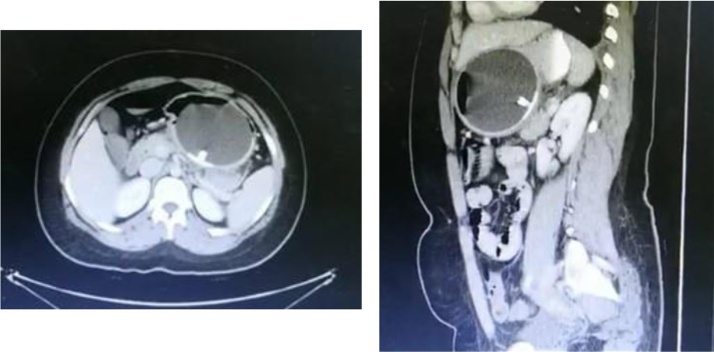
Computed tomography scan demonstrating compression of the pancreas by the intragastric balloon, obtained in case 1.
